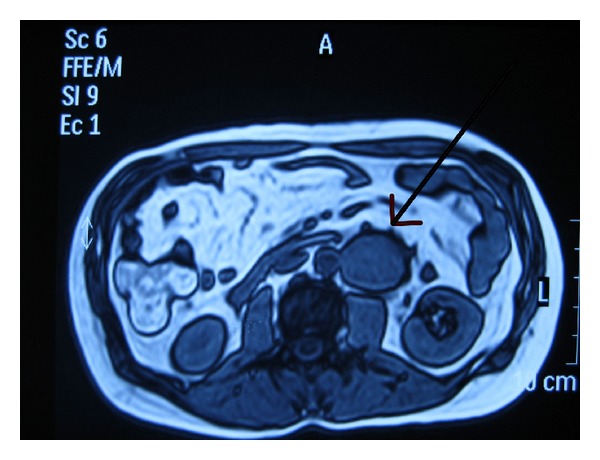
Abdominal MRI imaging depicting the lesion.
